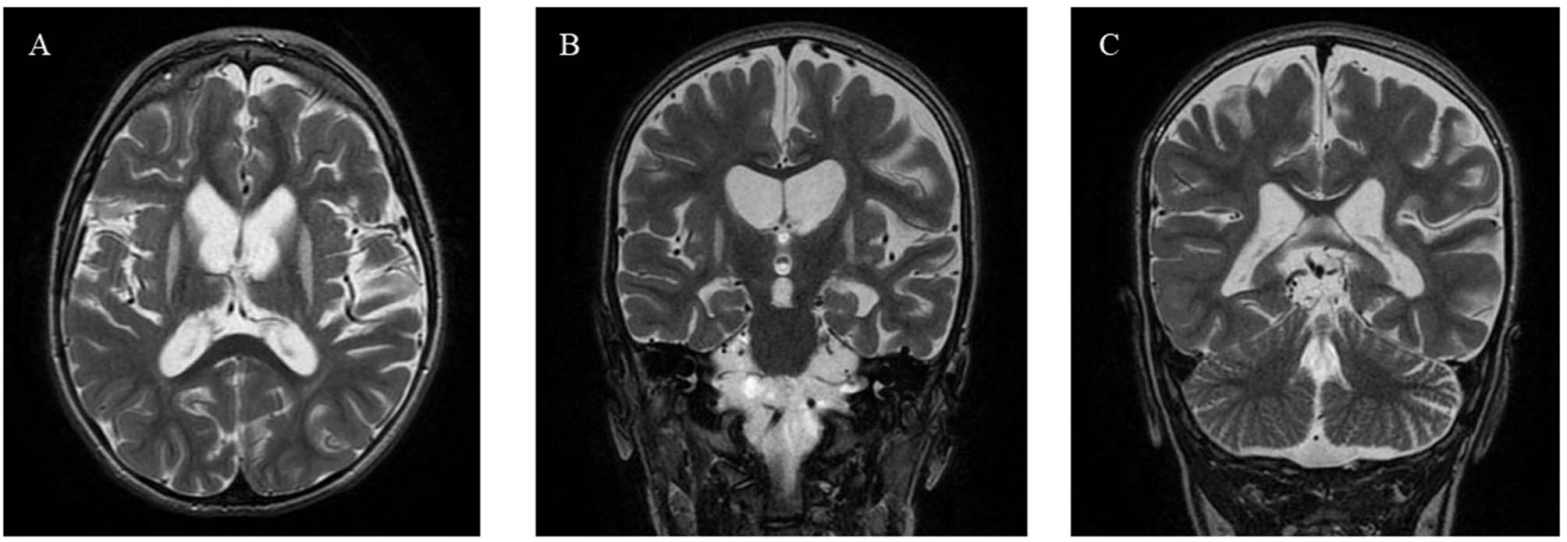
Magnetic Resonance Imaging (MRI) performed at 4 years of age in Patient 2 revealing parenchymal depletion with enlargement of subarachnoid spaces (A), alterations in the putamen, striatum, and caudate nuclei (B, C)
